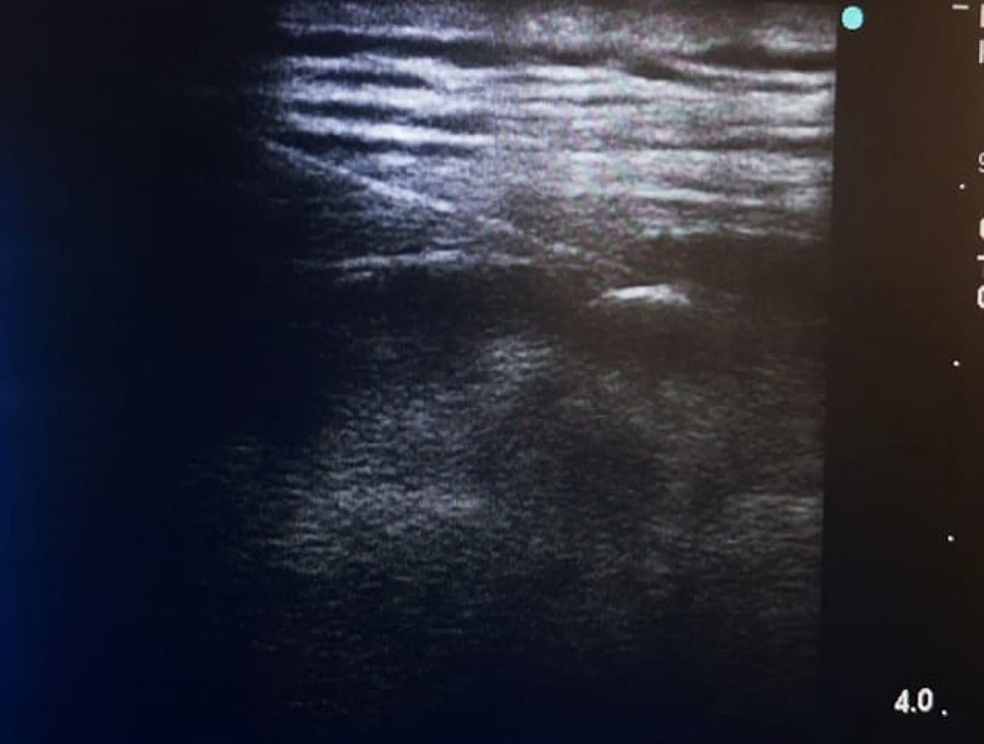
USG image of the erector spinae plane block application USG: ultrasonography
